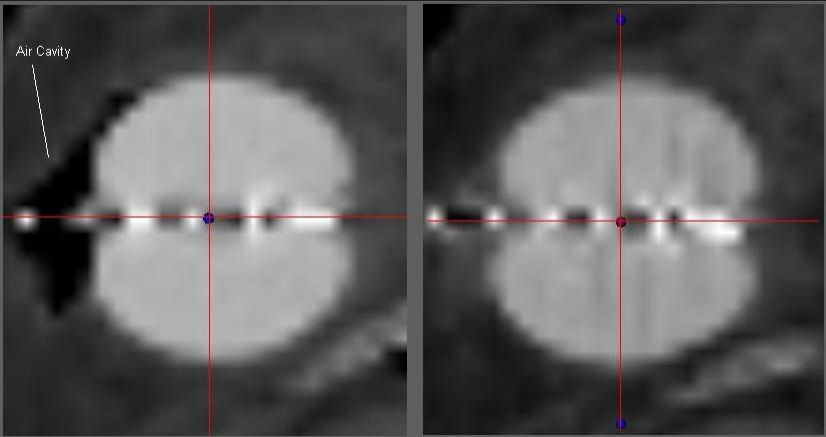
Pre‐ and post‐manipulation images of patient 5. The images are multi‐planar reconstructions of the computed tomography scan of the implant. The image on the left is the implant in its postoperative state. The image on the right is the implant after addition of 10 cm^3^ fluid to the balloon and massage of the implant area. The air pocket has been eliminated, with an acceptable 0.5 mm increase in asymmetry.
